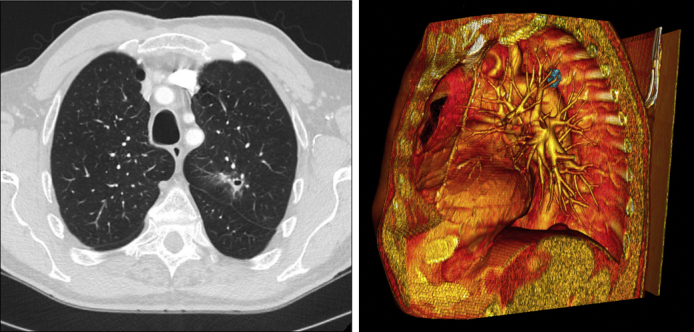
Two-dimensional computed tomography of nodule and 3D reconstruction of patent LITA graft and nodule (blue).
